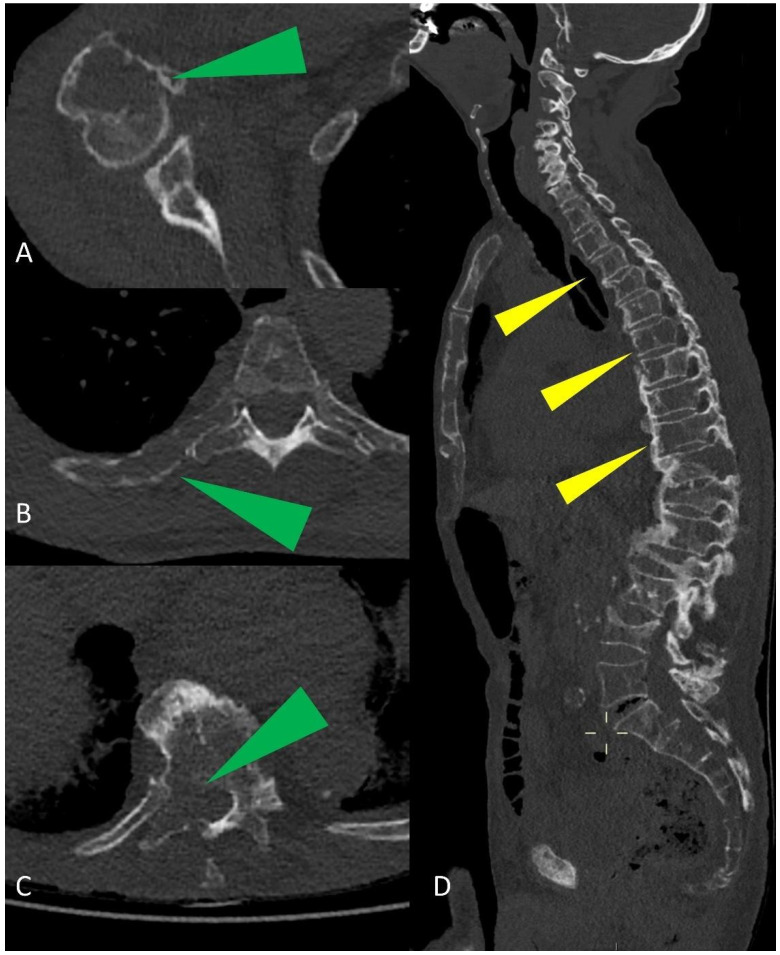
Multiplanar CT images showing humeral, ribs, and vertebral osteolytic lesions (green arrowheads in (A–C)); multiple osteolytic lesions of the spine (yellow arrowheads in (D)).
